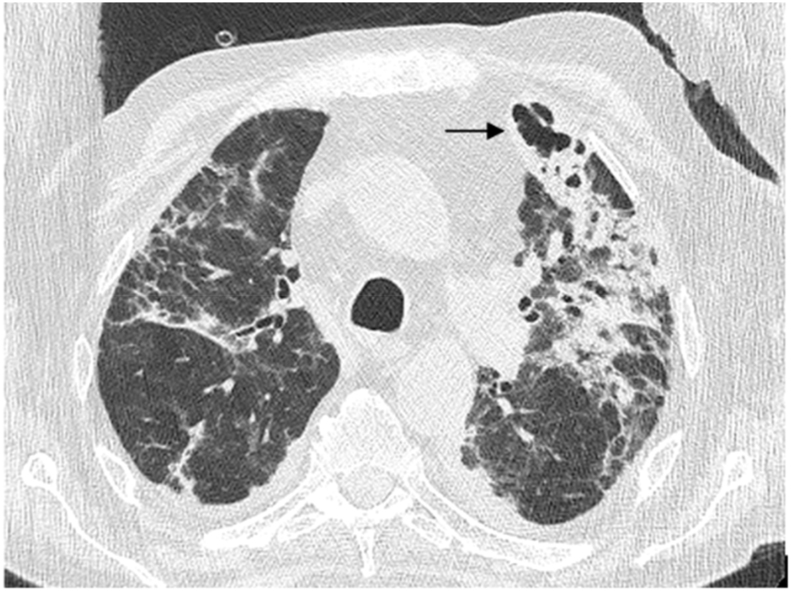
A chest CT showing cryptogenic organizing pneumonia and a subpleural cavitated lesion in the left upper lobe (black arrow).
